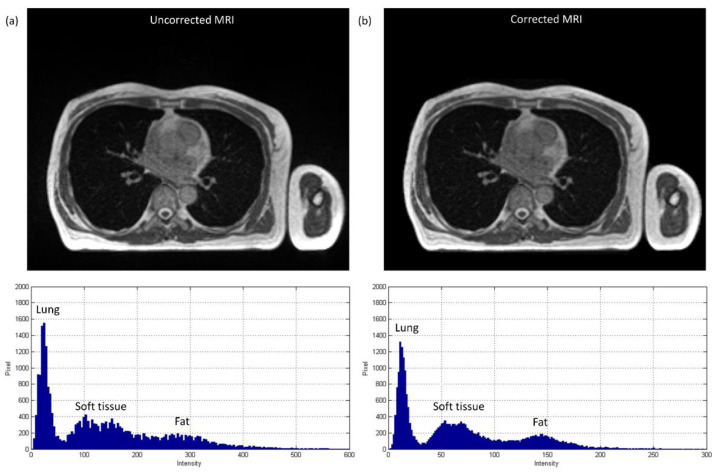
Axial image and histogram of (a) uncorrected MRI (WL/WW = 200/400) and (b) corrected MRI (WL/WW = 100/200) from 0.35 T scanner for chest imaging divided into CNN testing dataset (${\mathrm{IMG}}_{\mathrm{testing}}^{\mathrm{chest}}$).
